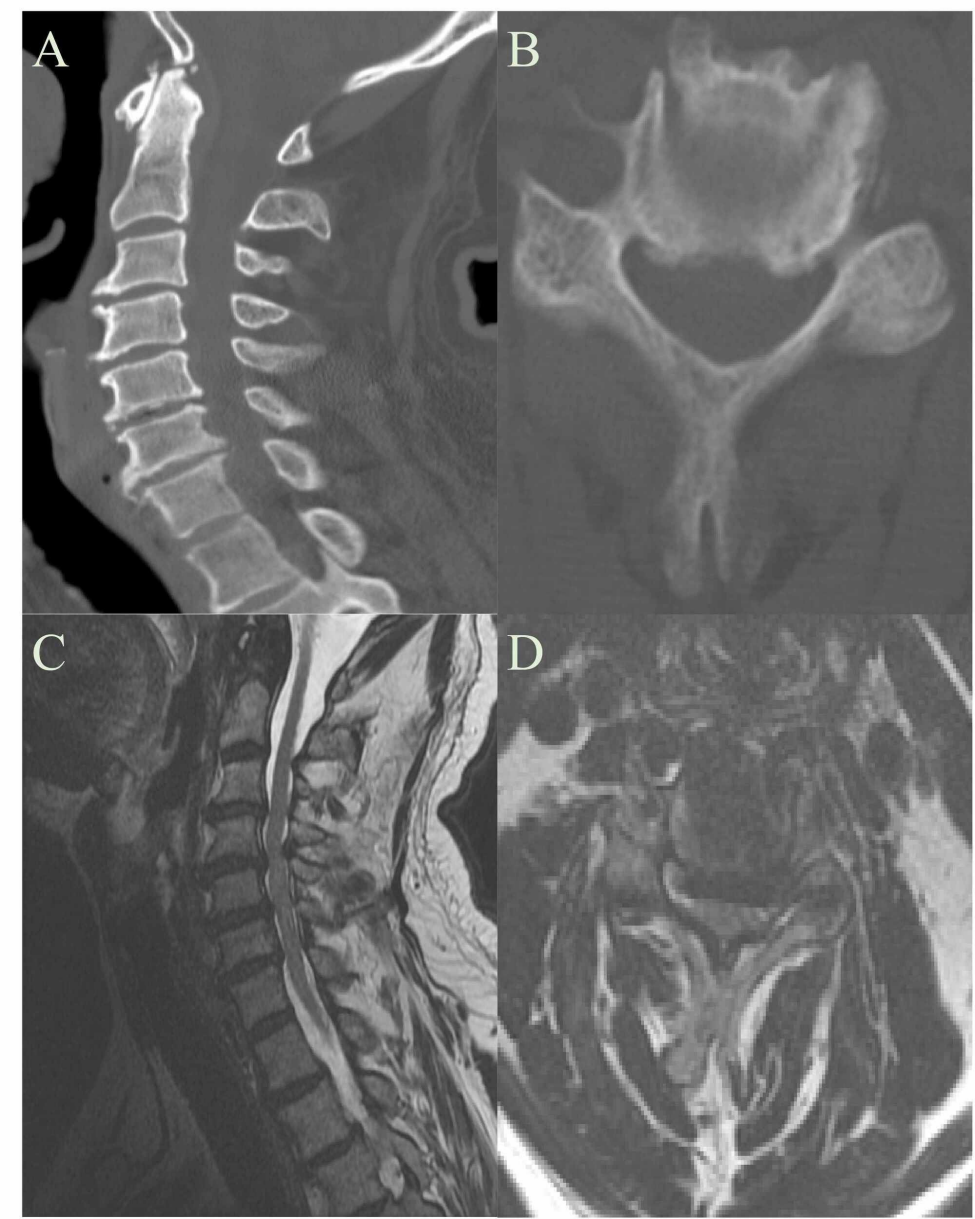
Patient 3 CT and MRI of the Neck A: Patient 3 CT Neck Sagittal View B: Patient 3 CT Neck Axial View C: Patient 3 MRI Neck Sagittal View D: Patient 3 MRI Neck Axial View Multilevel cervical stenosis can be seen in A and C.
